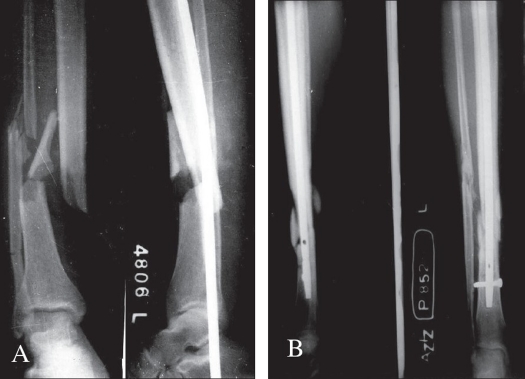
A) Radiograph (anteroposterior and lateral) of a 26-year-old male who sustained Type IIIB comminuted fracture both bone leg. Interlocked nail was done 11 hours after injury. B) Radiograph of the same patient after four months, showing union in progress
